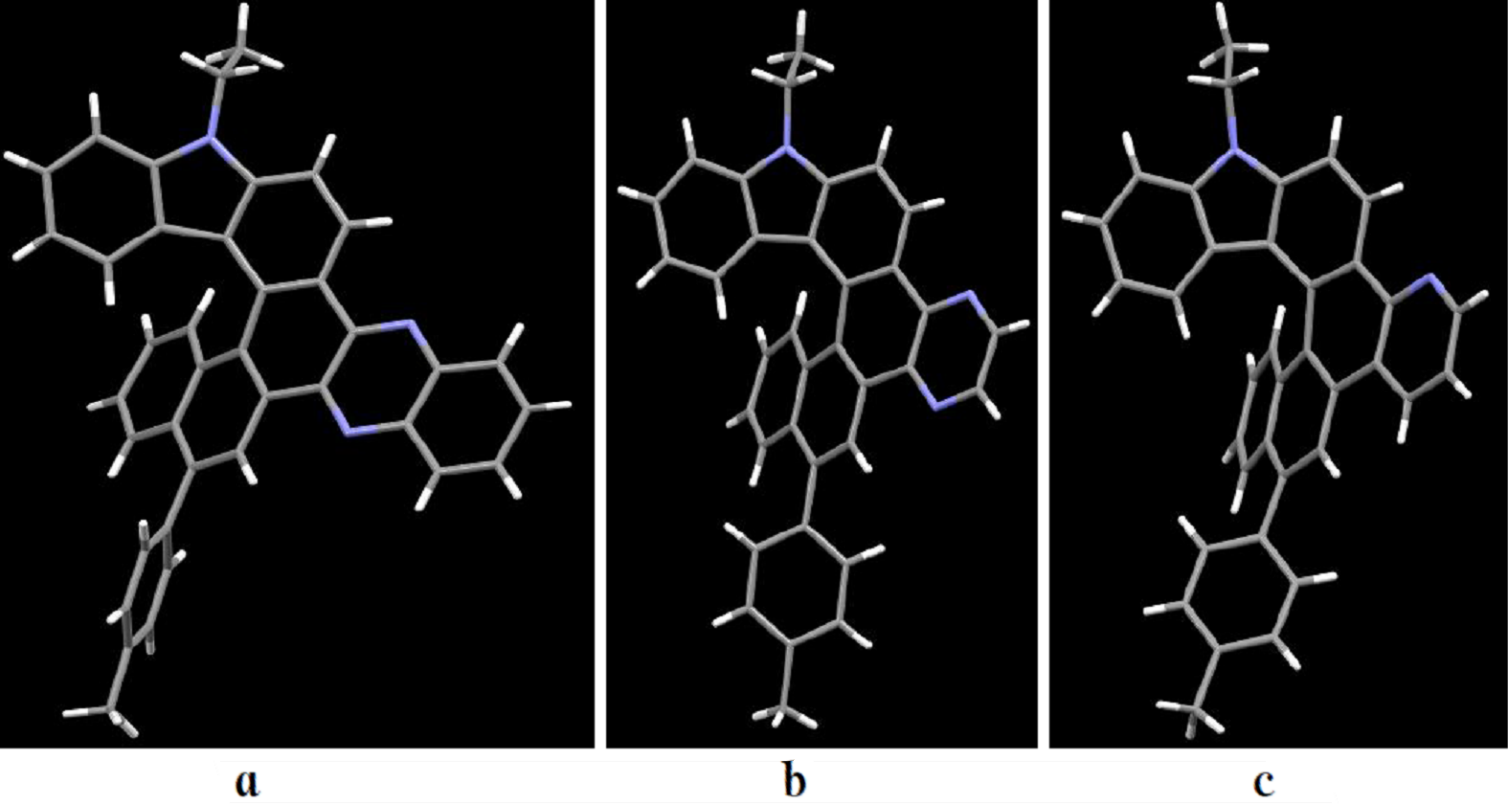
Molecular structure of carbazole-based [6]helicenes 10a (a), 10b (b) and 10c (c) (X-ray data).
